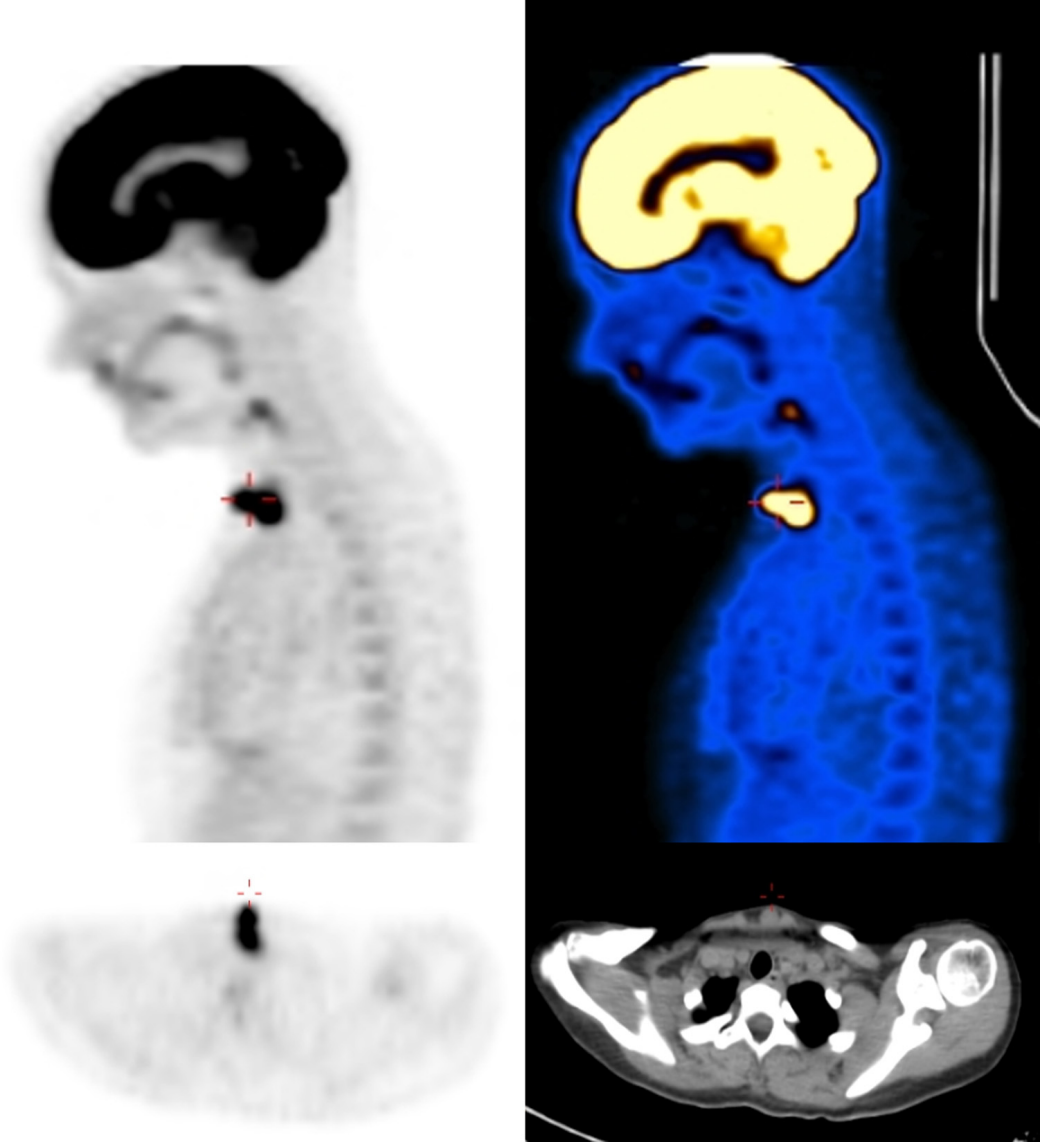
PET‐CT which was performed 6 months after the first mandibulectomy, and prior to last operation. A lump in the central neck, at the site of the tracheostomy scar, is noted. The lump shows FDG‐positive uptake.
